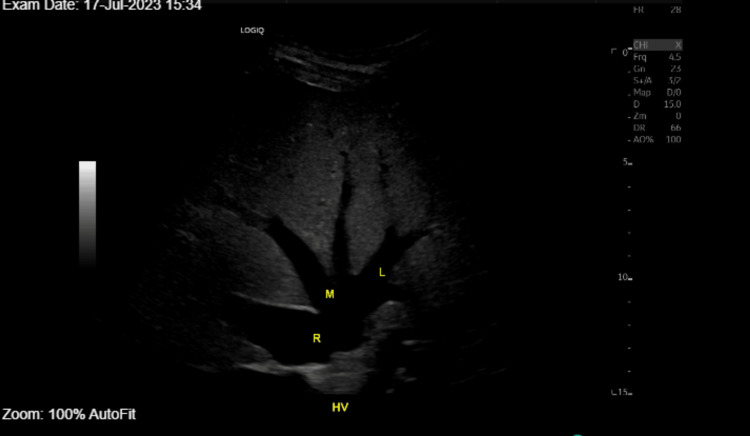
Abdominal liver US with patent IVC, common iliac veins, and hepatic veins, with no evidence of thrombosis. US: ultrasound, IVC: inferior vena cava; HV: hepatic vein; R: right; M: middle; L: left
